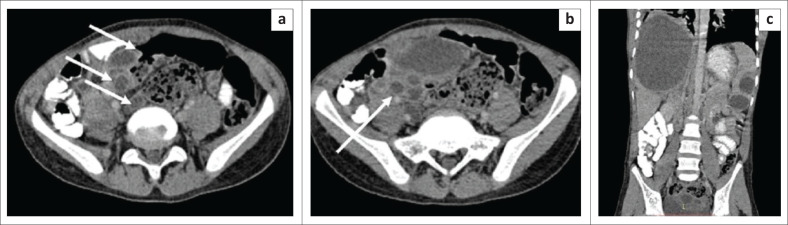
Hydatid cysts. Axial contrast enhanced CT images (a, b) of the abdomen in a 6-year-old child reveal multiple, well-defined peripherally enhancing unilocular cystic lesions in the mesentery and pelvis (white arrows) with no internal septations, mural nodule or calcification within the lesions. Coronal reformatted CT image (c) reveals well-defined unilocular cysts in the liver and spleen with no internal septations, calcifications or mural nodule.
